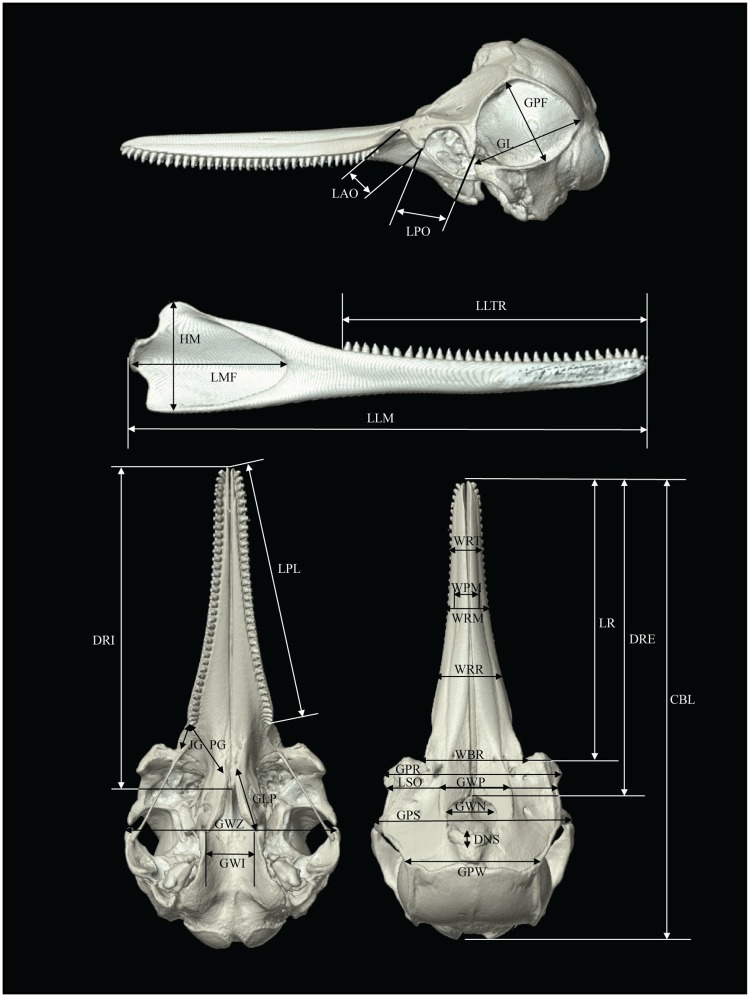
Key to cranial measurements made on CT 3DVRI of *Sousa chinensis*. For abbreviations, refer to Table 1.
